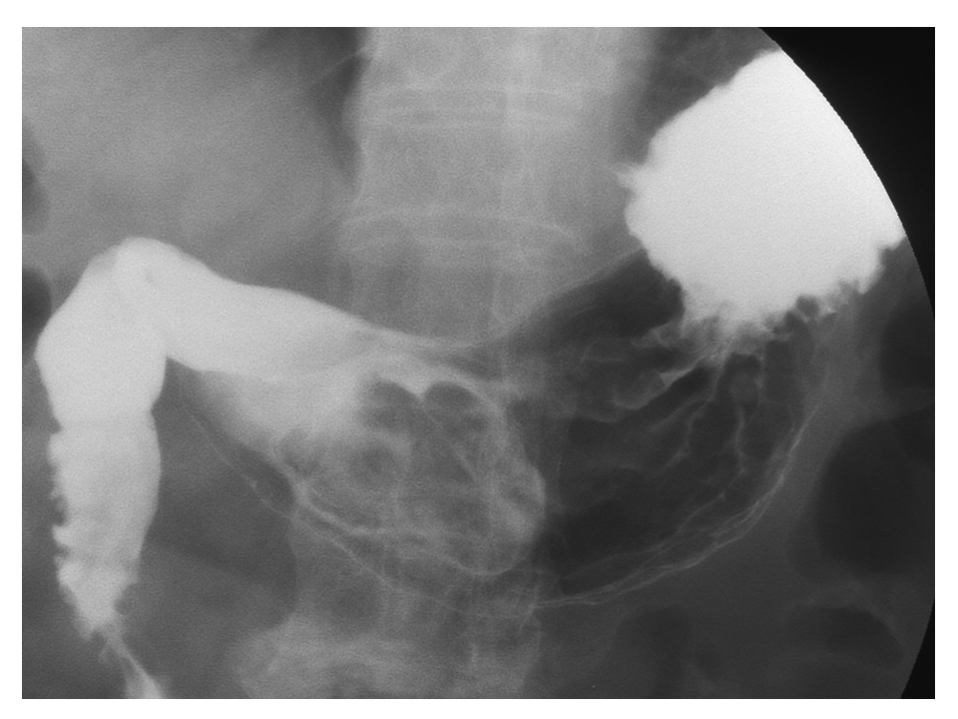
The major axis of the bezoar was found to be 7 cm by X-ray examination.
